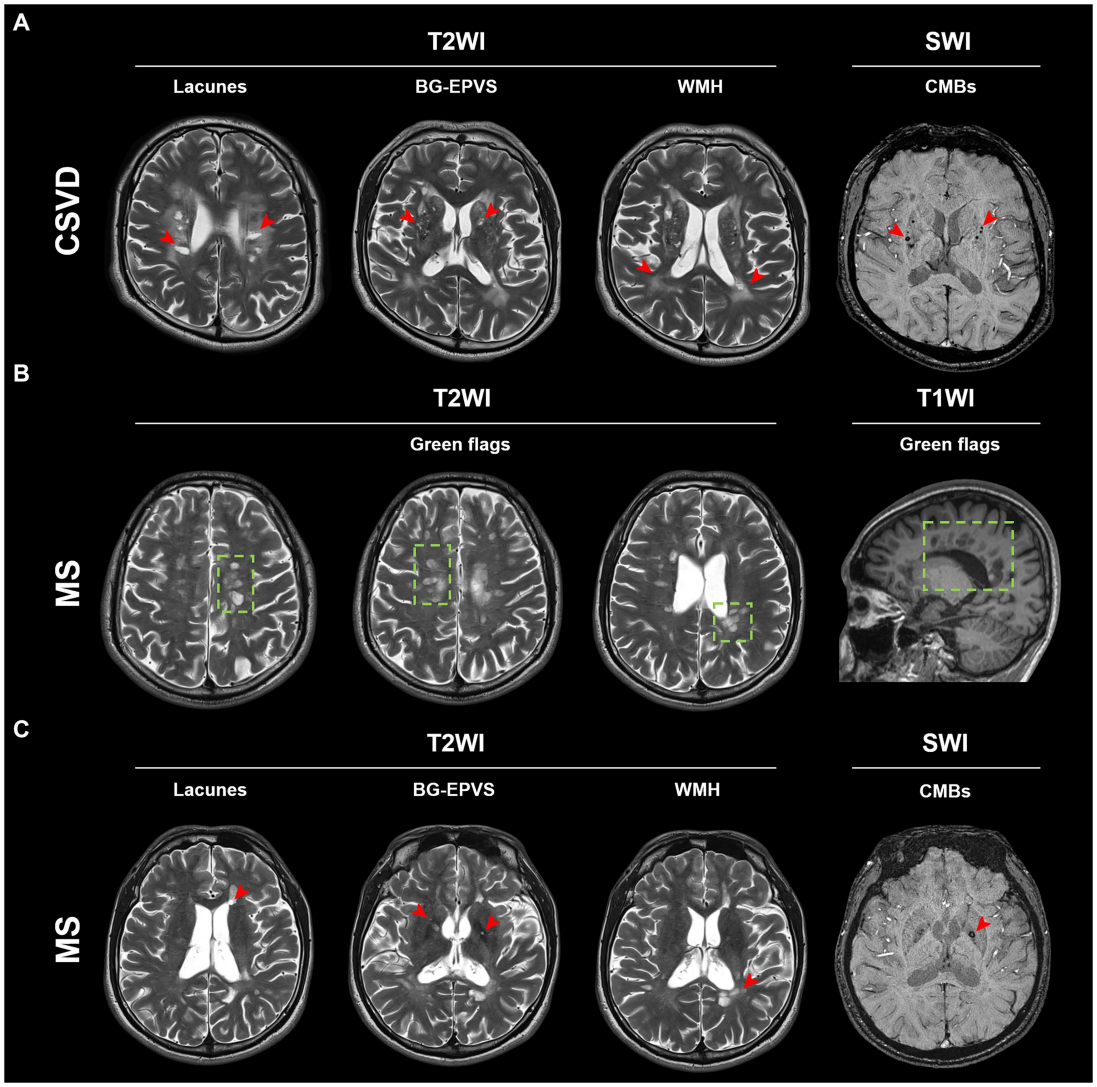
Typical MRI imaging features of MS and CSVD. (A) Typical lesions of lacunes, BG-EPVS, WMH, and CMBs (red arrow) in a CSVD patient. (B) Typical green flags in an MS patient. Green flags: periventricular lesions suggestive of multiple sclerosis; or periventricular lesions perpendicular to the corpus callosum (“Dawson’s fingers”). (C) Lacunes, BG-EPVS, WMH, and CMBs (red arrow) can also be found in this MS patient. BG-EPVS, Enlarged perivascular spaces (EPVS) in the basal ganglia; WMH, White matter hyperintensity; CMBs, Cerebral microbleeds; MS, Multiple sclerosis; and CSVD, Cerebral small vessel disease.
